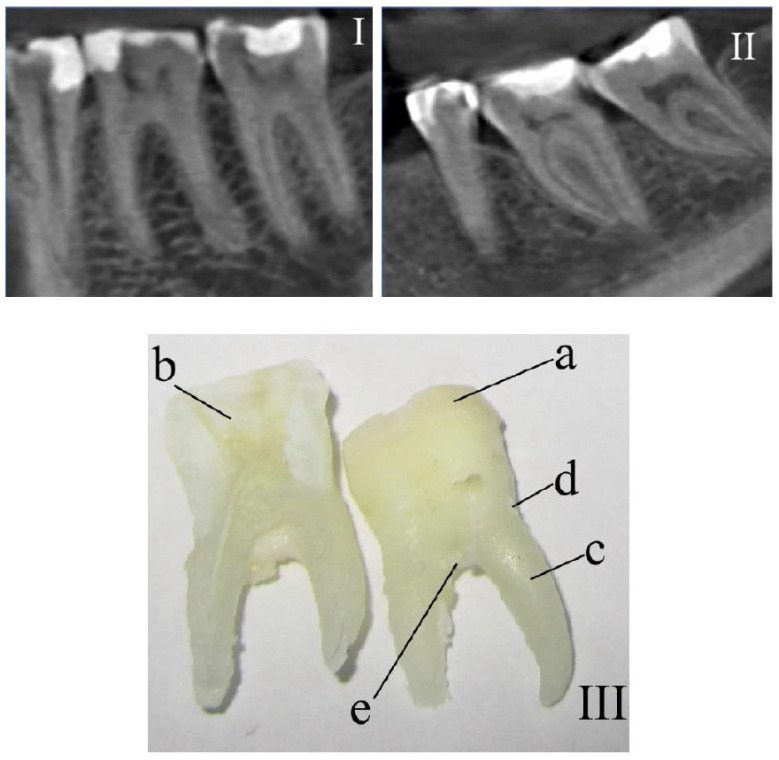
The fragments of teeth following computed tomography that were (I) healthy and (II) diagnosed with periodontitis. (III) Photo of a tooth with the researched areas indicated: a—enamel, b—dentin, c—cementum, d—above-gum dental calculus, and e—under-gum dental calculus.
